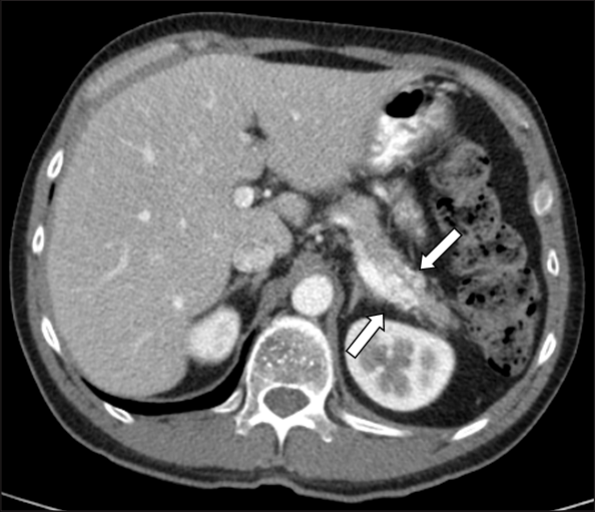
Contrast-enhanced axial CT scan (February 2012) showed a hypervascular lesion (white arrows) in the pancreatic tail in proximity to the splenic vein and measuring 2.5 cm × 2.4 cm × 1.7 cm.
